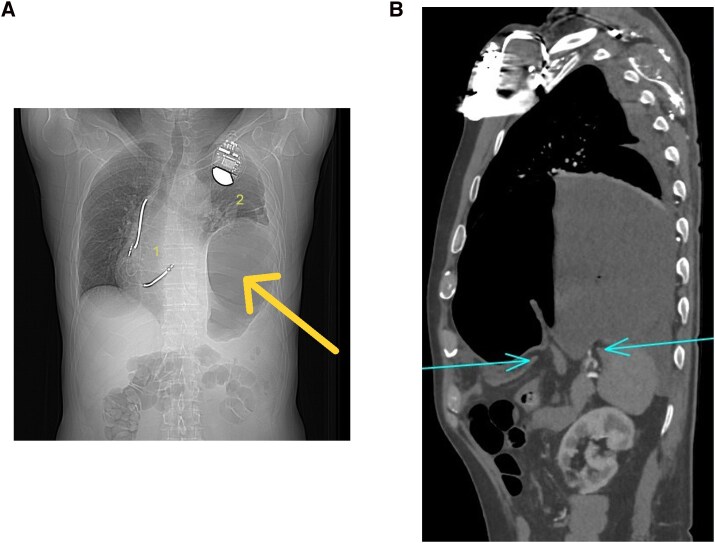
(*A*) Anteroposterior computed tomography scout view with the herniation of abdominal organs into the left thoracic cavity (yellow arrow), displacing the heart (1) towards right and the left lung (2) superiorly. (*B*) Sagittal computed tomography view with blue arrows marking the hernial opening, and abdominal organs extending into the left thoracic cavity.
